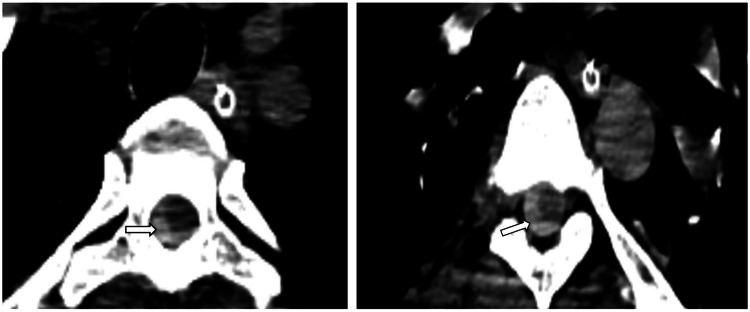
Axial non-contrast CT image showing epidural hemorrhage in the dorsal spine at D5-D6 and D6-D7 levels (arrows).
